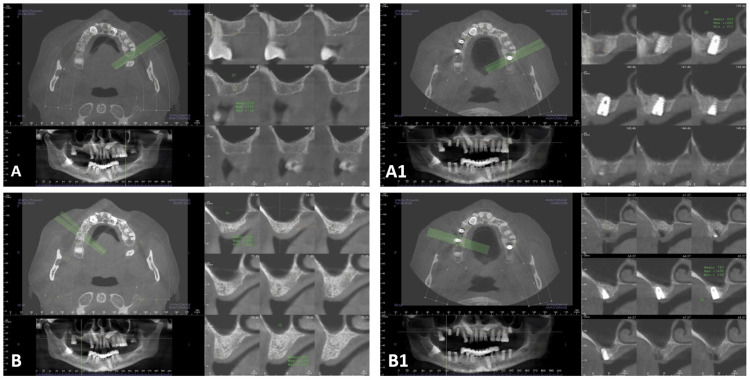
Pre and Post-op CBCT for T-PRF and A-PRF (A) CBCT baseline reading for T-PRF; (A1) CBCT 3-month follow-up reading for T-PRF. (B) CBCT baseline reading for A-PRF; (B1) CBCT 3-month follow-up reading for A-PRF. CBCT, Cone-Beam Computed Tomography; A-PRF, Advanced Platelet-Rich Fibrin; T-PRF, Titanium Platelet-Rich Fibrin
